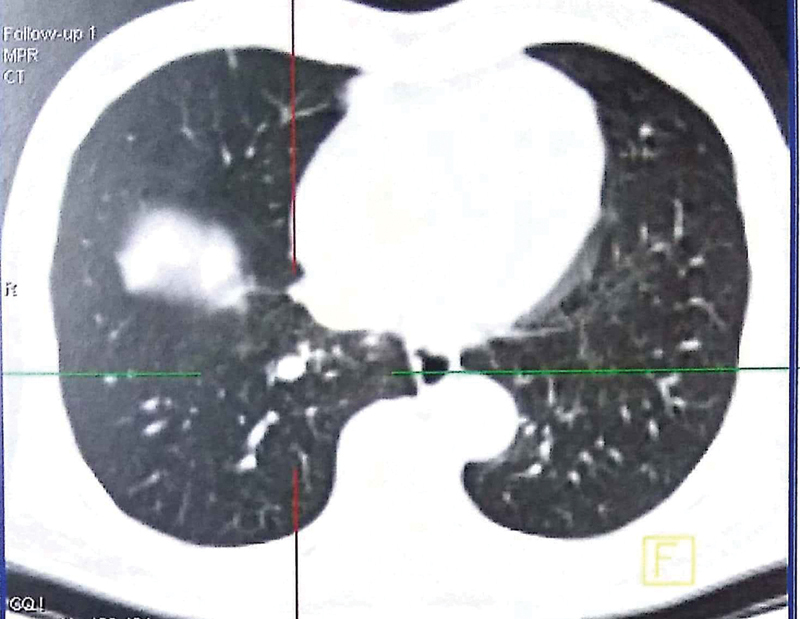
Positron emission tomography–computed tomography scan thorax shows resolution of lesions in left lung field. A single lesion is seen in the right lobe which has also reduced in size.
